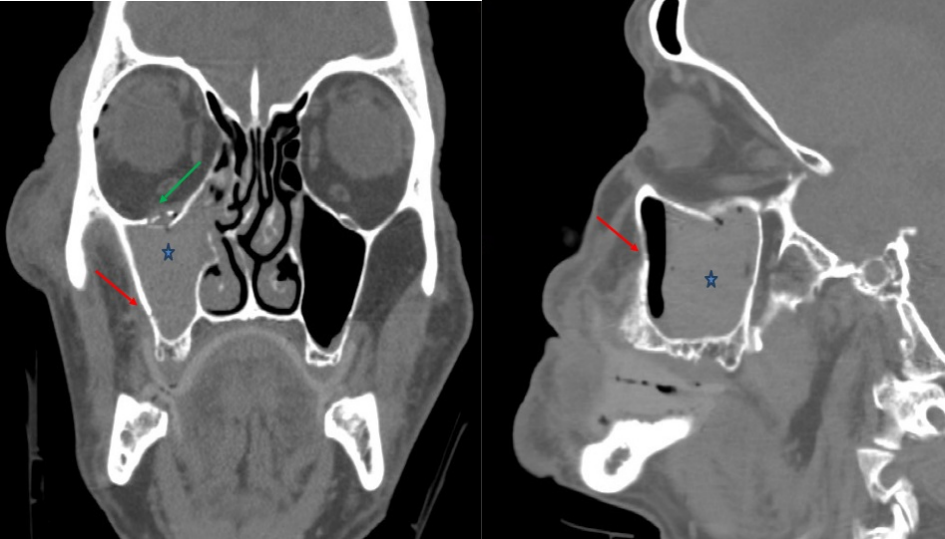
Example head CT image with maxillary sinus opacification Coronal (left) and sagittal (right) non-contrast head CT images. Example of a typical fracture involving the right orbital floor (green arrow) and medial maxillary sinus wall (red arrow), which is associated with resultant hemorrhage and an air-fluid level in the right maxillary sinus (blue star).
